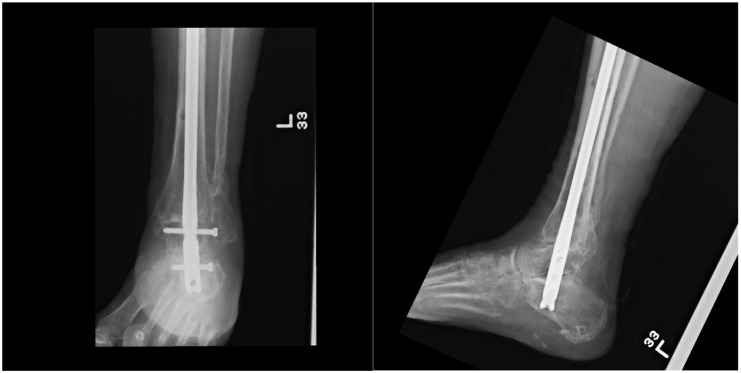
Representative postoperative radiographs showing tibiotalocalcaneal (TTC) fusion with a retrograde intramedullary nail. Anteroposterior and lateral views demonstrate hindfoot alignment and stable nail fixation across the ankle and subtalar joint.
